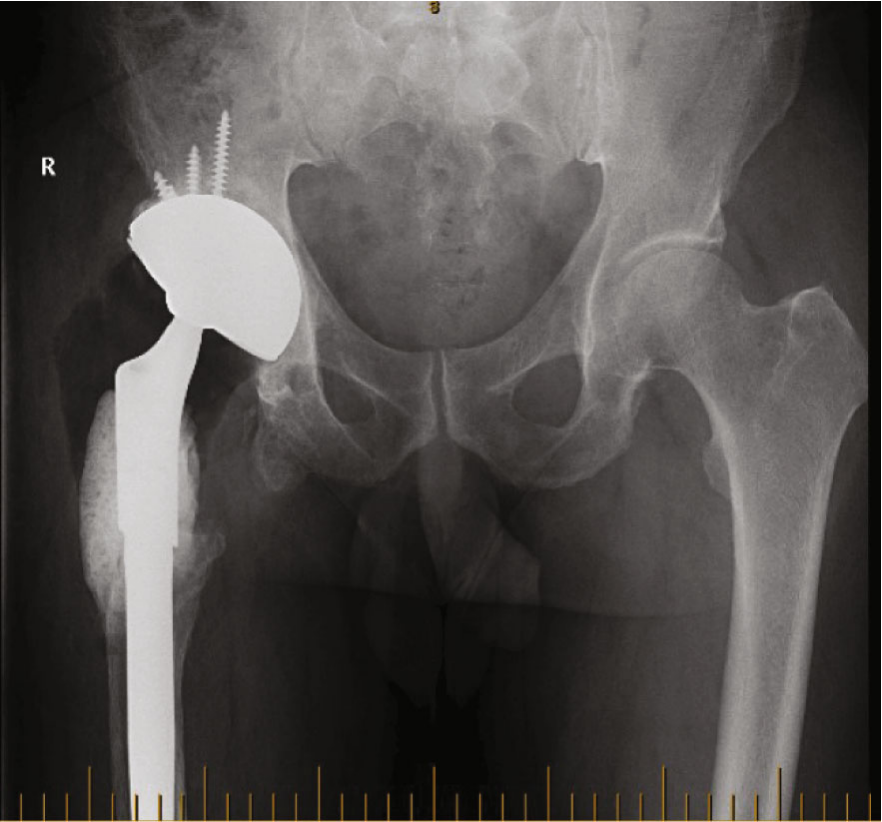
Pelvic X-ray 2017 post revision with the Inovaris prosthesis.
